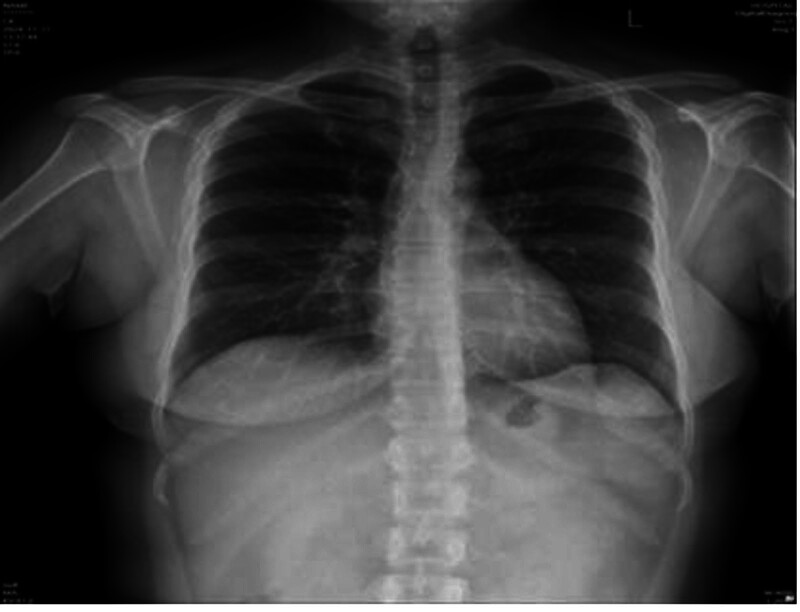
Chest x-ray no significant abnormalities.
